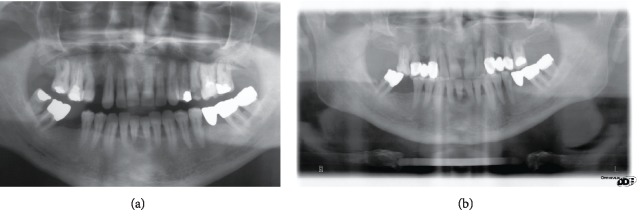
Panoramic radiographs of the patient exposed at year 2008 (a) and 2017 (b): loss of teeth and periodontal breakdown are visible.
